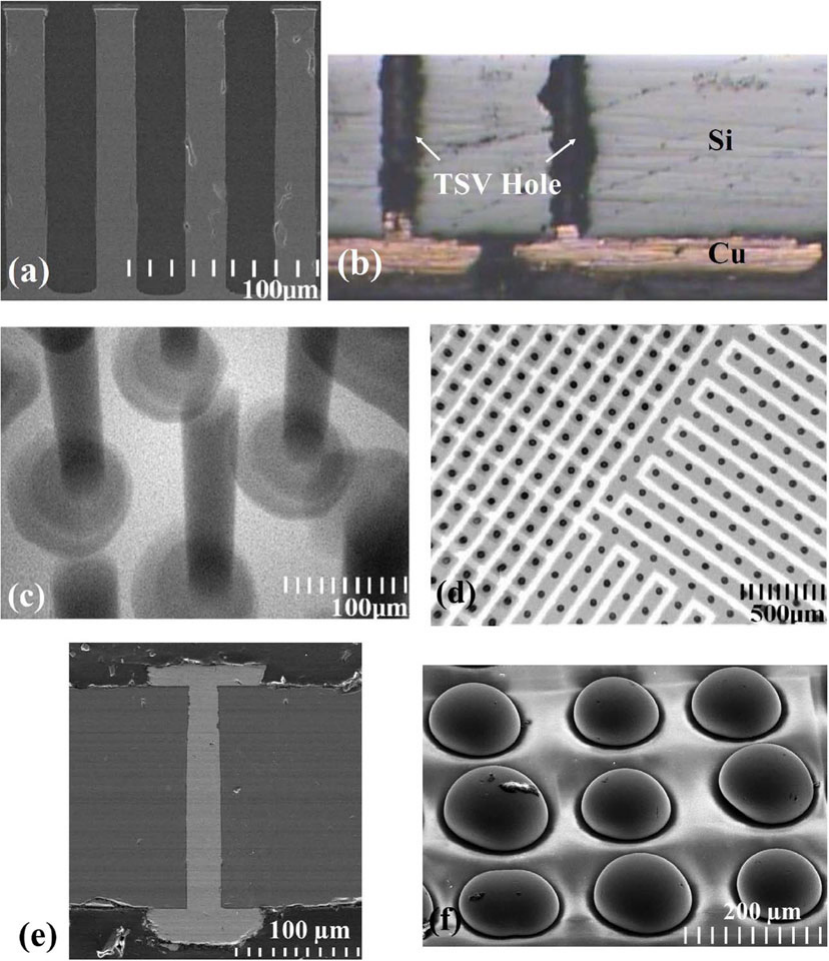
a Dry etching profile of 25 μm via. b Sealing bumps fabricate before TSV filling. c Void-free filled TSVs by X-ray inspection. d The *black dots* are Cu TSVs; the *white area* is the SiO2 region; the *gray-colored area* is the metal lines. e TSV cross section with Cu bumps on both sides. f Final structures of Cu bumps with TSVs [20]
